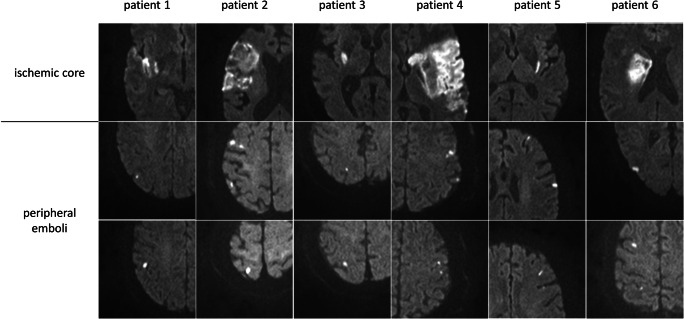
Example of six patients with ischemic cores (upper row) and peripheral emboli (lower rows) on the trace images of the high-resolution DWI
